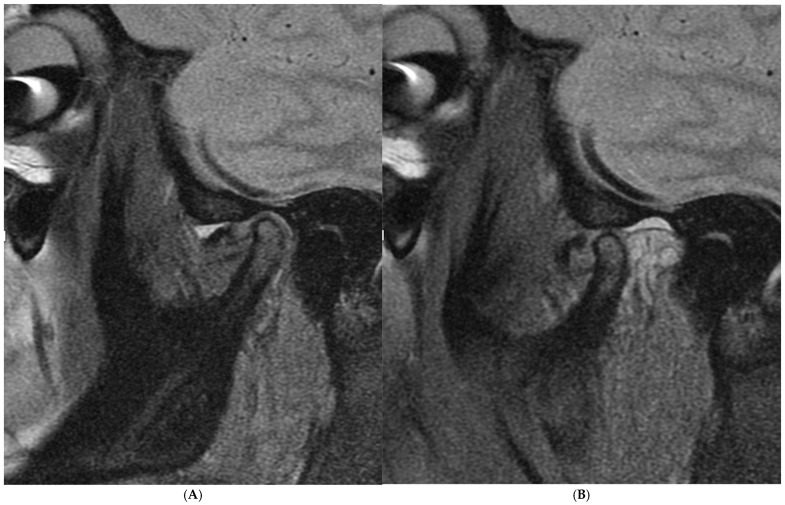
The picture shows an anterior disc dislocation with the mouth closed (A) and open (B) in MRI.
